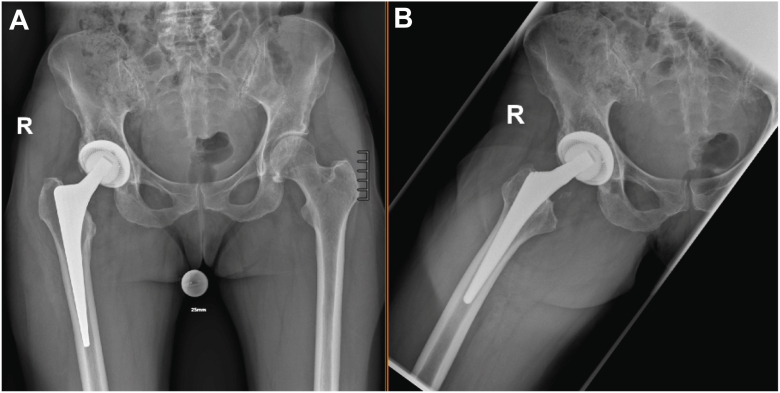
X-rays at the initial presentation in January 2024 showing no evidence of loosening or fracture of the right hip prosthesis. (a) Anterior-posterior pelvic view. (b) Axial view of the right hip according to Lauenstein.
